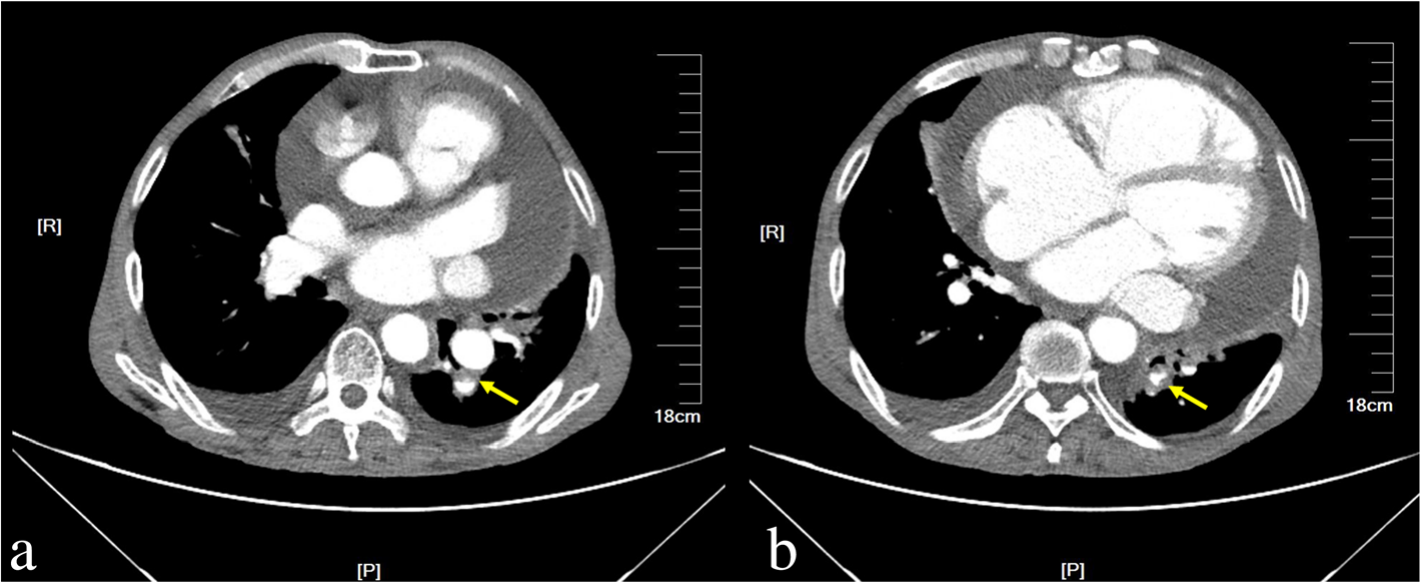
Emergency thoracic contrast enhanced computed tomography revealed pulmonary embolism. a, b filling defects in the branches of the left lower pulmonary artery (yellow arrow)
